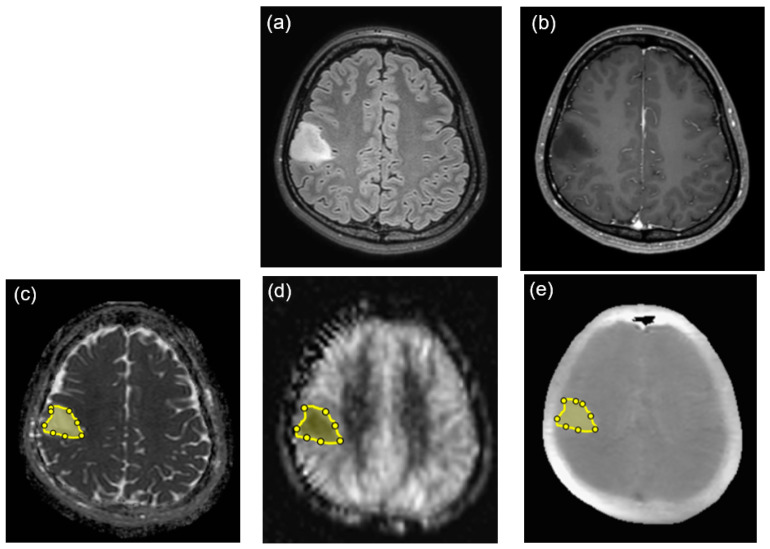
Segmentation of the tumors on the right parietal lobe. The solid portion of the tumor showing a hyperintense signal on FLAIR (a) and no enhancement on contrast-enhanced T1WI (b) were manually segmented on the ADC map ((c), yellow). The segmented region of interest was copied from the ADC map of the software to the pCASL image ((d), yellow) and APT image ((e), yellow) of the software.
